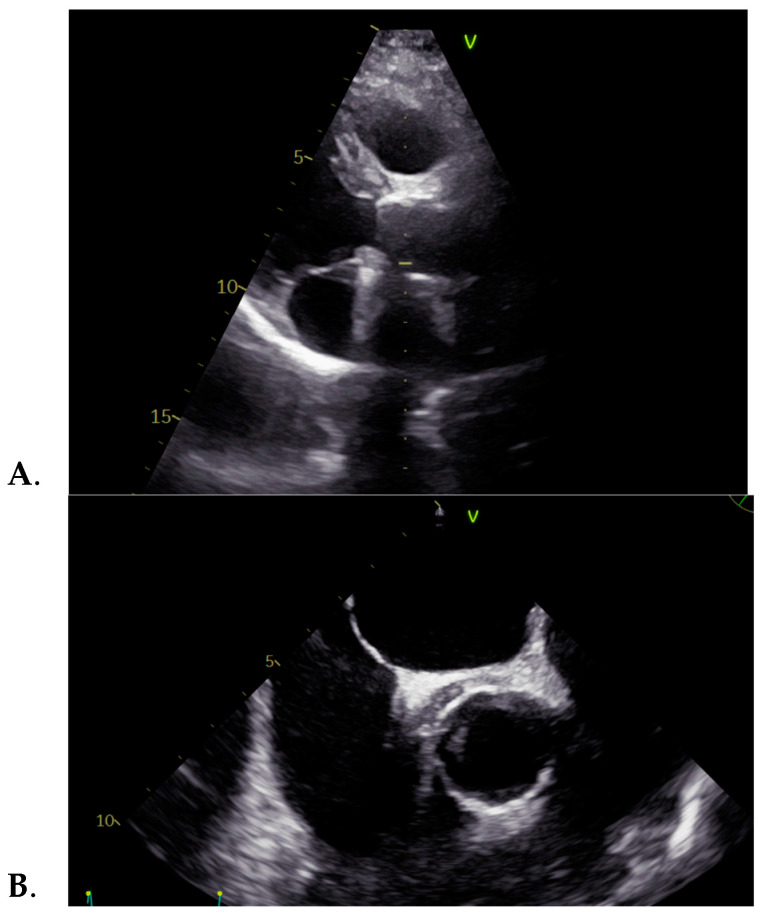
(A) Transthoracic and (B) transesophageal echocardiographies showing an echo-dense mass surrounding the ascending aorta.
